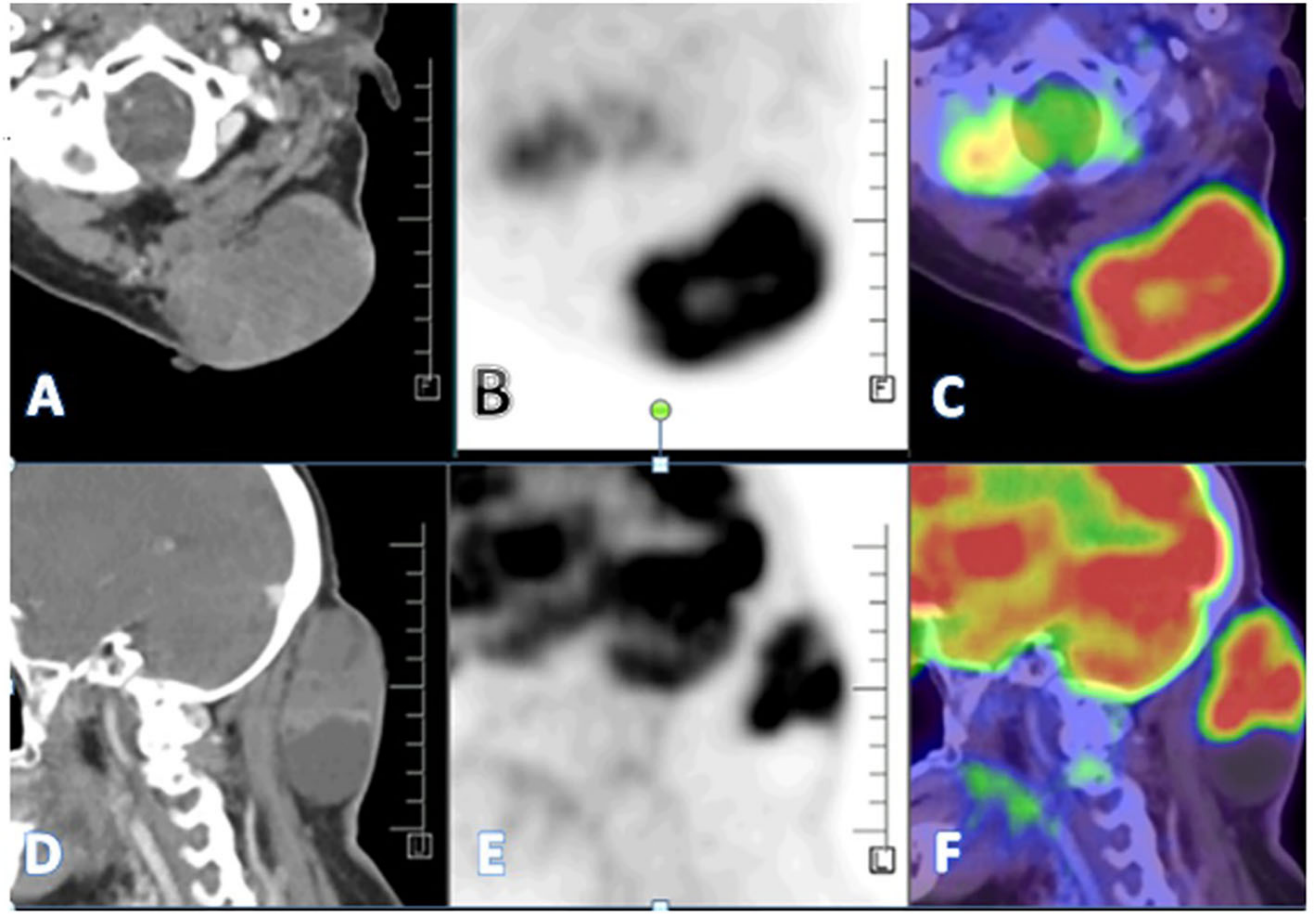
(A–F) - A large heterogeneously enhancing FDG avid (SUV max 11.8) solid cystic lesion seen in the left paramedian aspect of the nape of the neck measuring 67 x 41 x 75 mm involving overlying skin and closely abutting the underlying muscles-likely malignant.
